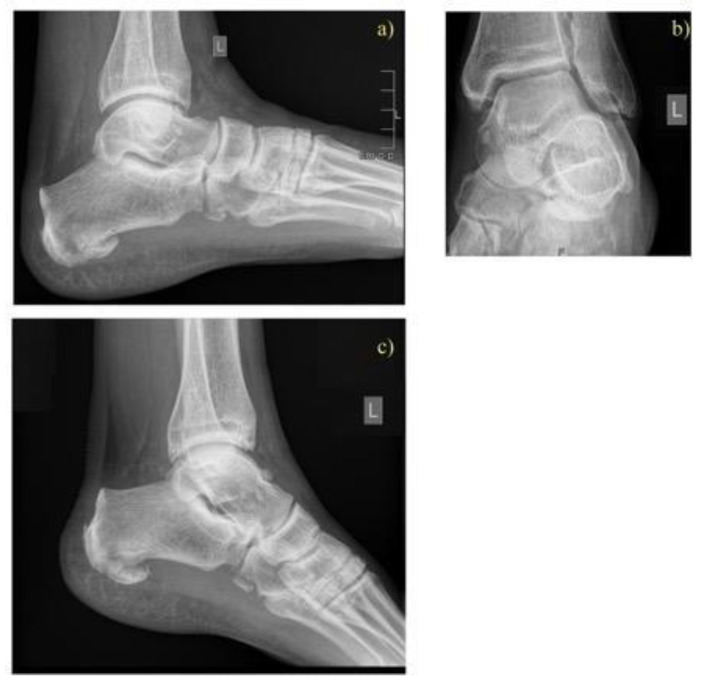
Conservative case: 43-year-old patient with bilateral calcaneal fracture after a fall. The left foot showed a slight dislocation of a fracture fragment (Sander type II) on X-ray (a,b). Follow-up after 10 months (c).
